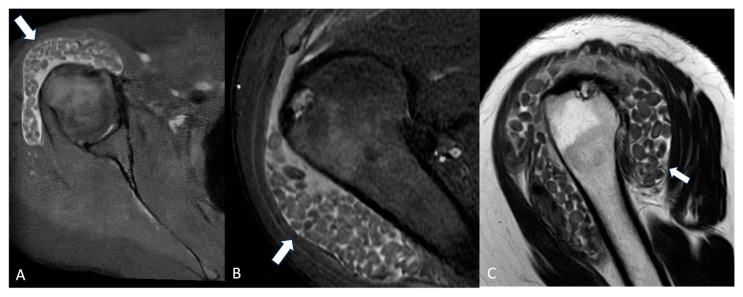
Axial PD-FS (A), coronal PD-FS (B), and T2-weighted (C) MRI images of a 48-year-old female patient presenting with shoulder pain and swelling. Multiple non-osseous cartilaginous loose bodies (arrows) are visible within the bursa, which appears enlarged, causing bulging of the deltoid muscle. Findings are consistent with synovial chondromatosis.
